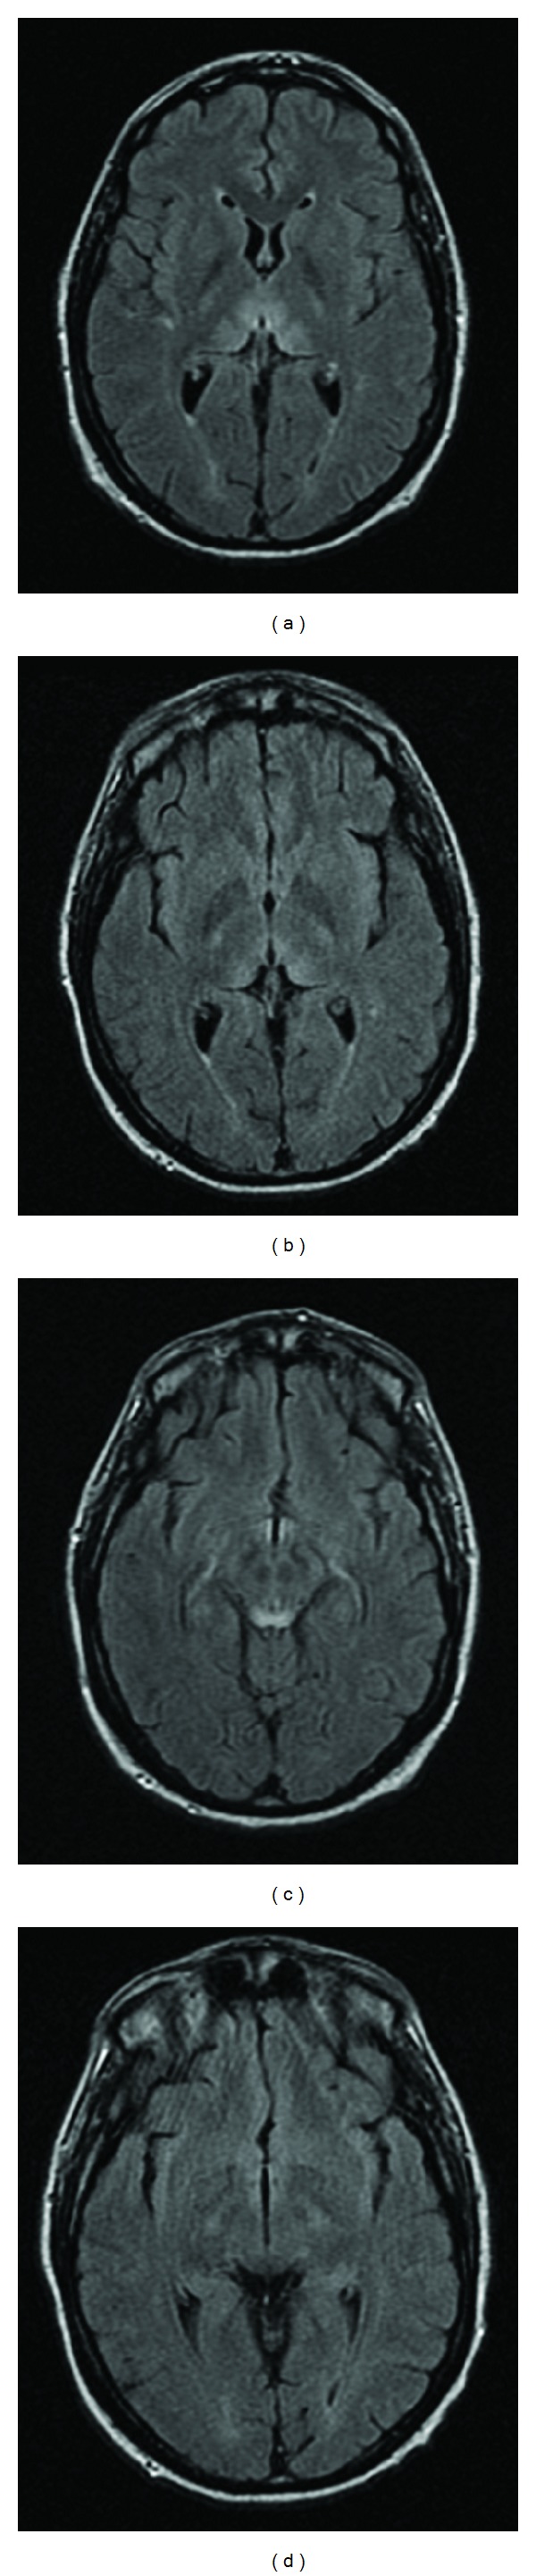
Corresponding FLAIR MRIs one day ((a), (c)) and seven days ((b), (d)) after admission. Signal abnormalities in bilateral thalami (a) and interval improvement (b). Signal abnormalities involving the hypothalamus (c), periaqueductal gray matter (c), and interval improvement (d).
